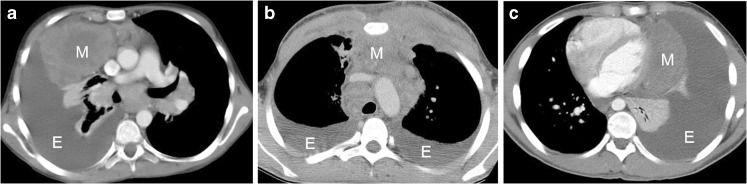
Axial CT images of mediastinal masses and associated pleural effusions. Most patients with eccentrically positioned masses toward one hemithorax or the other and moderate or large effusions demonstrated a much higher likelihood of only ipsilateral effusions (a, c), while a more centrally positioned large mediastinal adenopathy presents with bilateral effusions (b). a A 16-year-old girl; (b) another 16-year-old girl; (c) a 7-year-old boy. *E* effusion, *M* mass
